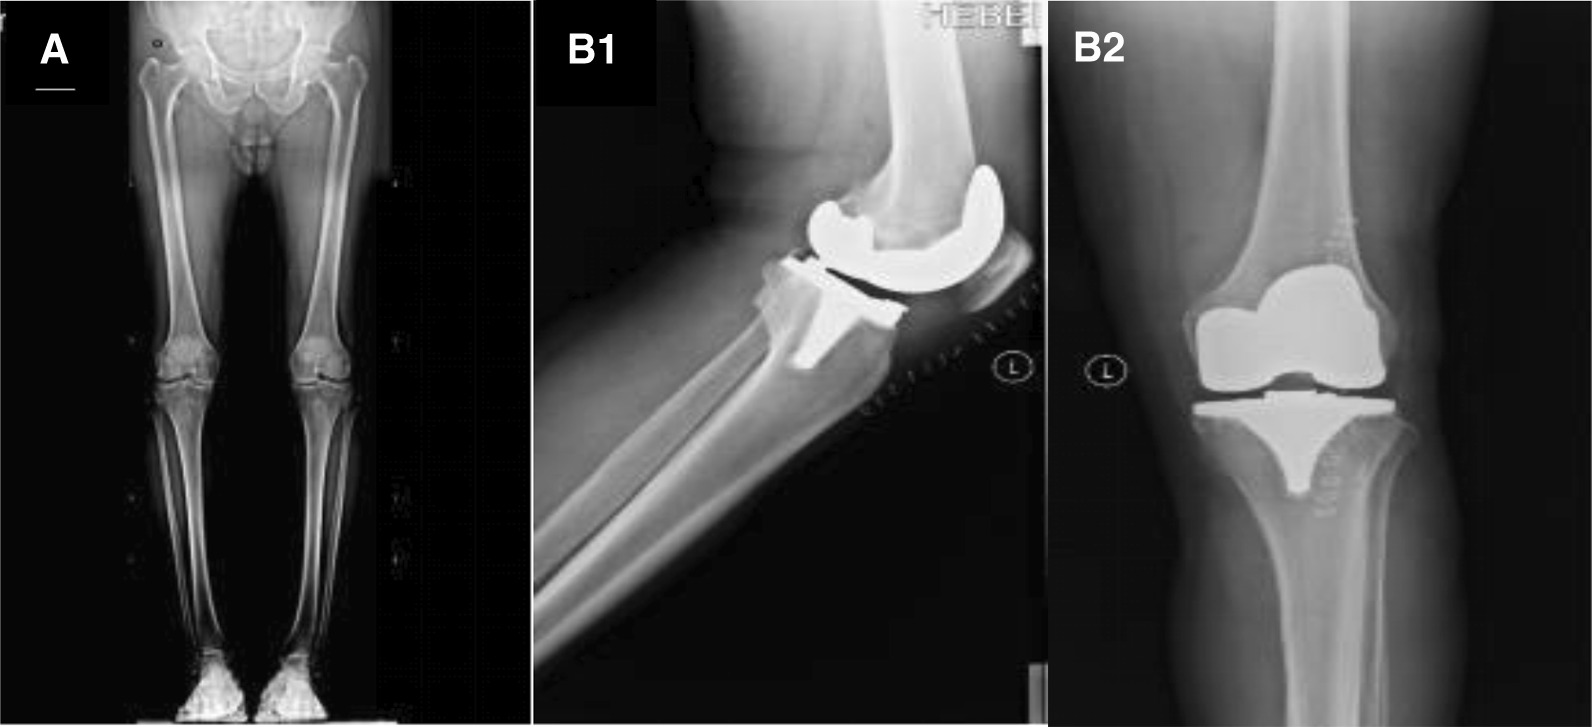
A, B X-ray findings before and after surgery. A Preoperative X—ray showed the knee osteoarthritis. B Positive X-ray (B1) and lateral X-ray (B2) examination at 1 day after operation showed the image performance after knee replacement
